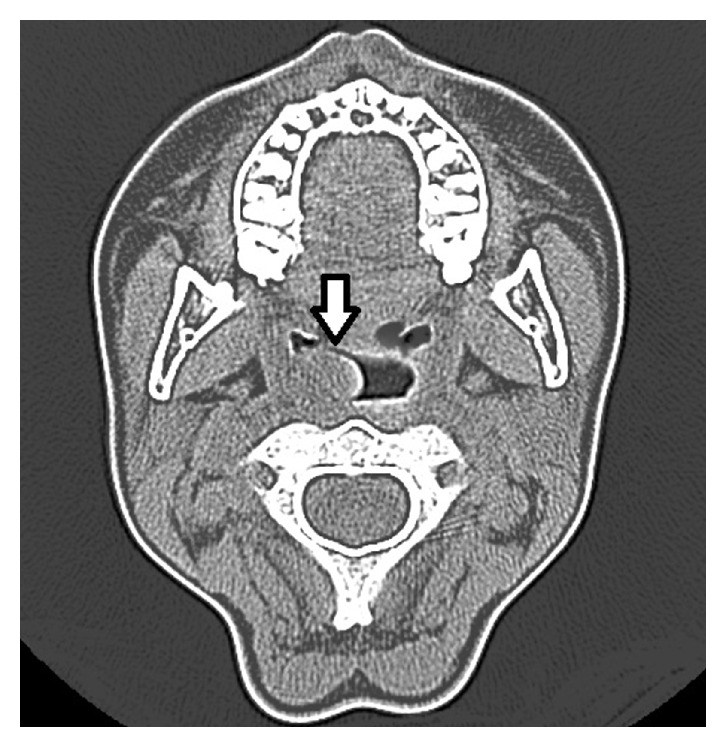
Preoperative axial neck CT.
